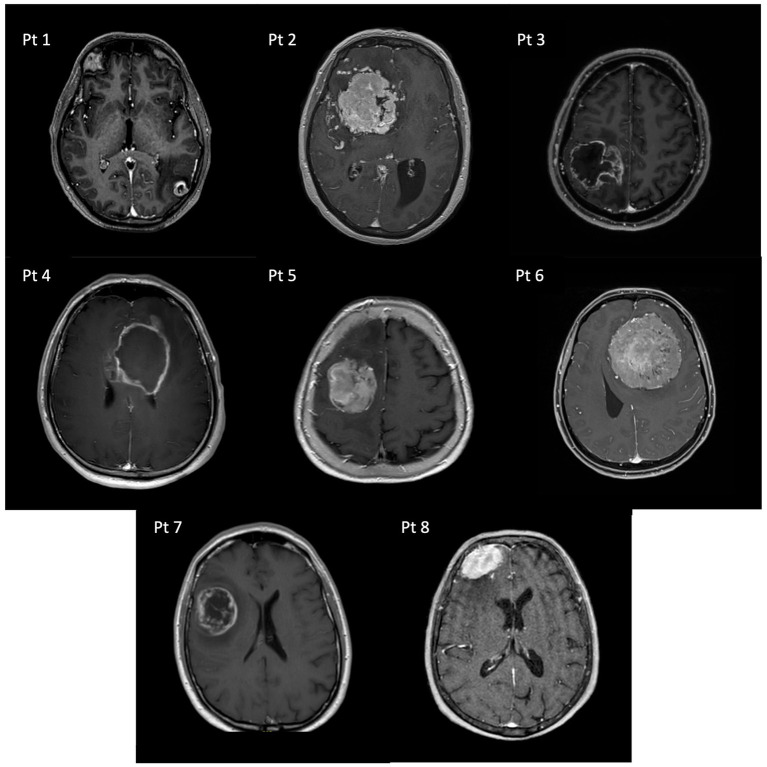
Pre-operative axial T1-weighted MRI scans of patients with impaired FC.
